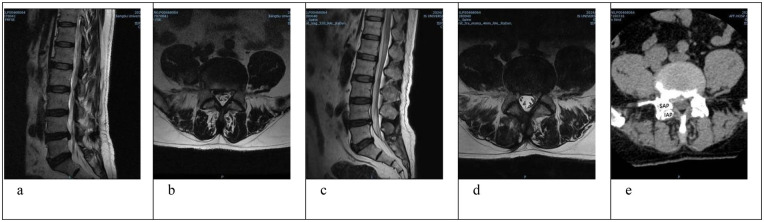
A 59-year-old male patient. (a) Preoperative sagittal lumbar MRI showing an L4/5 disc herniation. (b) Preoperative axial lumbar MRI shows a right paracentral disc herniation compressing the nerve root and dural sac. (c,d) One-year follow-up lumbar MRI shows complete removal of the herniated nucleus pulposus following UBE-assisted discectomy combined with annular suturing, adequate nerve root decompression, no recurrence of disc herniation, and no progression of disc degeneration. (e) Postoperative axial lumbar CT shows complete removal of the herniated nucleus pulposus and well-preserved facet joints.
